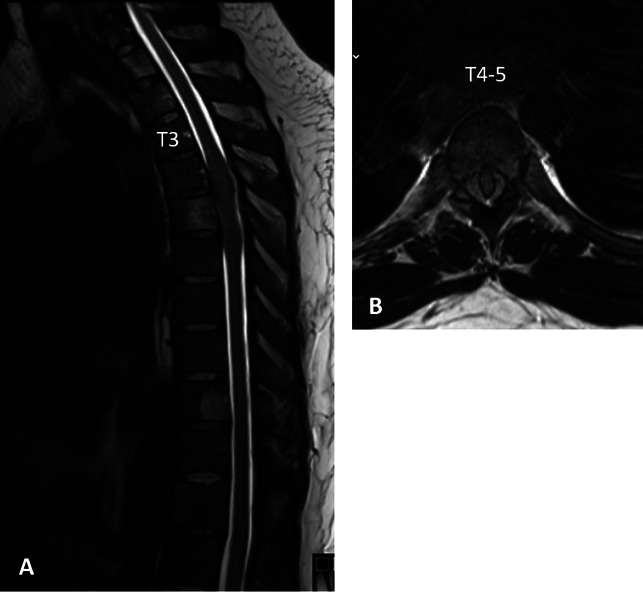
Radiographs showing sagittal T2 MRI (A) and axial cross section at the level of T4-5 (B) from the patient's initial presentation 5 years before recent presentation showing an epidural mass from T4-5.
